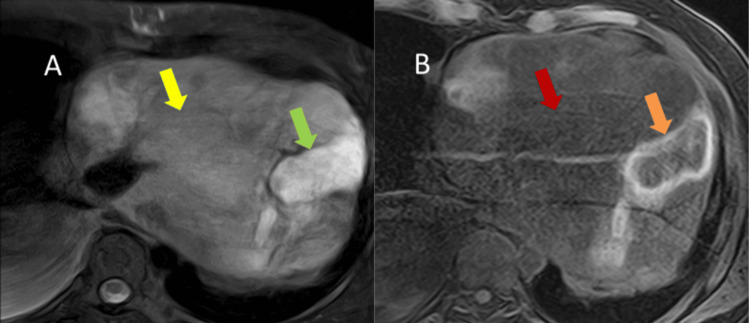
(A) Axial T2W MRI of the lower chest with a large pericardial mass appearing T2 hypointense (yellow arrow), with few areas of moderate T2 hyperintensity (green arrow). (B) Axial T1W post-contrast MRI of the lower chest with hypoenhancing mass (red arrow), with few areas of heterogeneous enhancement (orange arrow). T2W: T2-weighted, MRI: magnetic resonance imaging, T1W: T1-weighted
